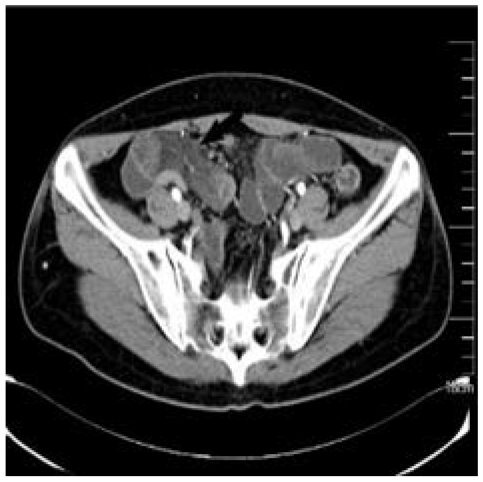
CT of patient B’s abdomen. Pelvic local intestinal wall edema, disorder, and mesenteric edema.
